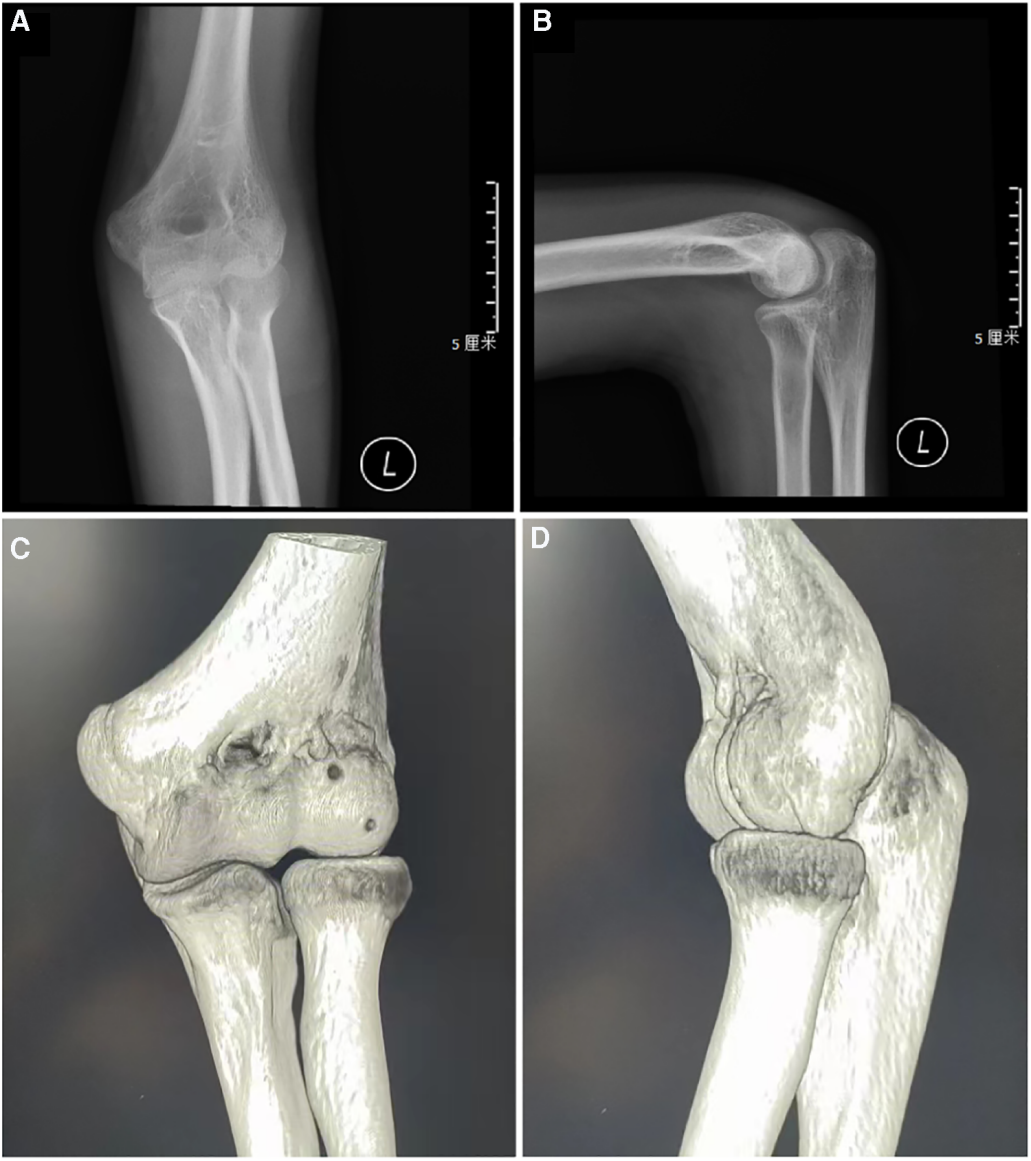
Follow-up: lateral (A) and AP radiographs (B) after surgery in 4-week later. CT images 2 months after surgery (C,D).
